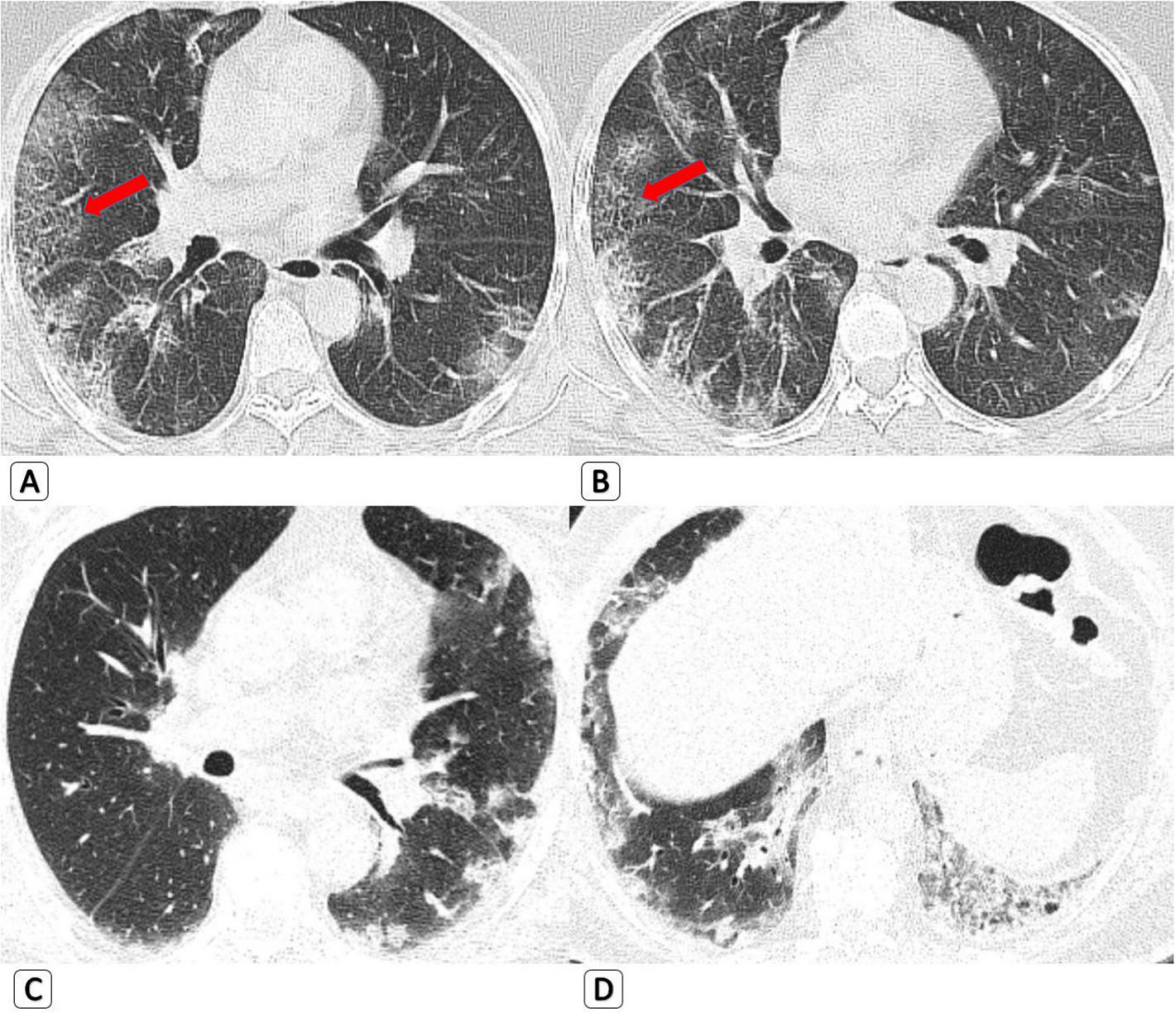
Pure parenchymal/alveolar pattern which cannot differentiate COVID19 from H1N1. a, b 44-year-old-male *COVID-19 patient* with axial HRCT chest lung window showing bilateral upper and lower lobar peripheral sub-pleural pure ground glass opacities. They shows smooth septal thickening on the right side “Crazy paving pattern” (red arrows). c, d 54-year-old male *H1N1 patient* with axial HRCT chest lung window showing bilateral upper and lower lobar mainly peripheral and sub-pleural dense ground glass patches
